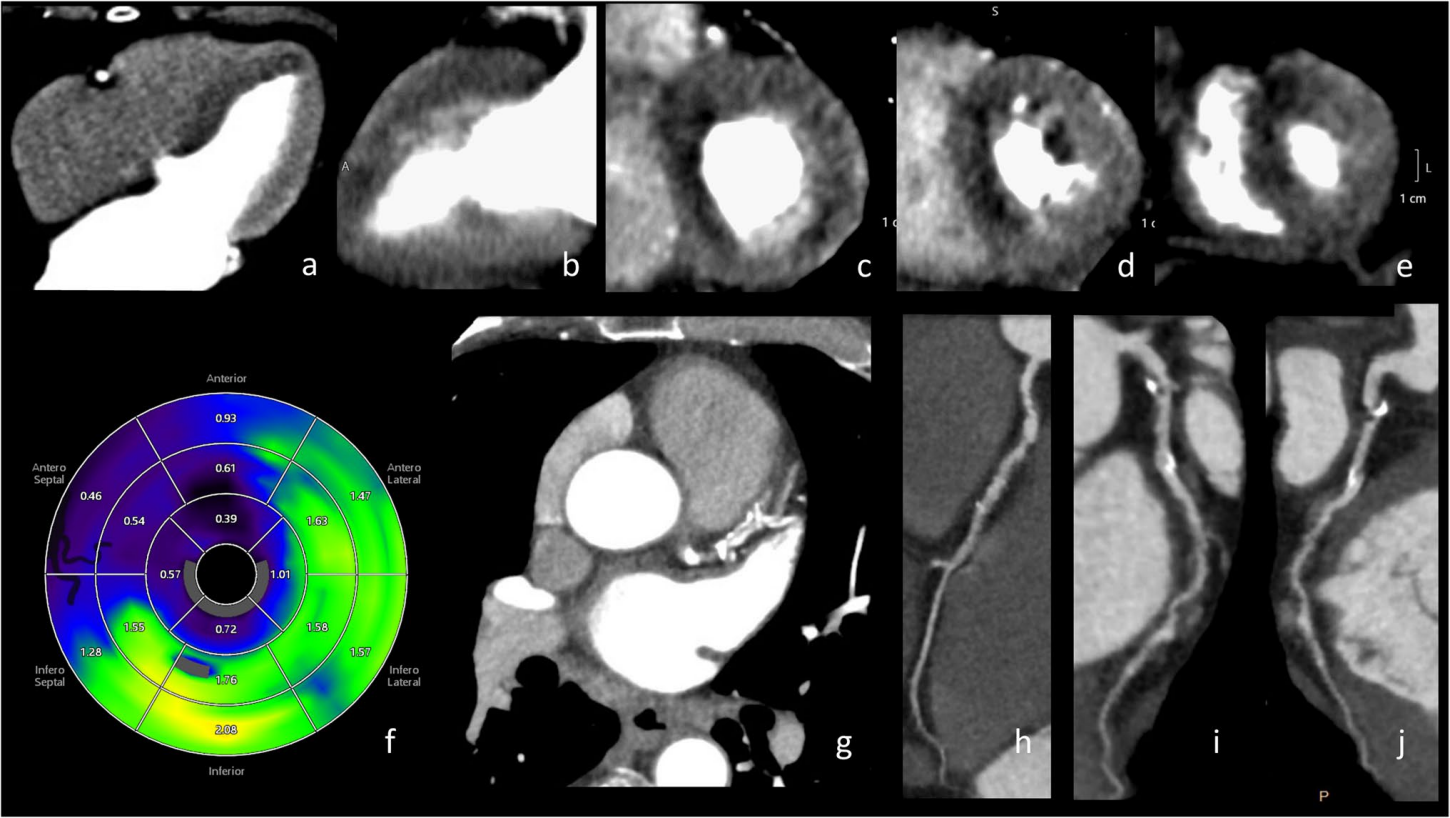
A 70-year-old male with poorly-regulated hypertension presented to the outpatient clinic with stable angina pectoris. Three months prior, he experienced chest pain, but acute coronary syndrome was excluded at the ER. The patient underwent a CT examination with calcium score, coronary CTA, and dynamic stress CTP protocol. The calcium score was 278.83 (62^nd^ percentile). Coronary CT angiography showed 50% stenosis in the distal left main artery (i), stenosis of 70–99% in the proximal (i), the mid-LAD (i), and origo LCX (j). In the proximal RCA (h), the intermediate stenosis based on the non-calcified plaque was 50%-69%. In the mid-LAD and mid-LCX: plaques with high-risk plaque features (positive remodeling and hypo-attenuated plaques). Positive adenosine stress CT perfusion with extensive ischemia in LAD territory in multiple segments (a–f). Furthermore, the ischemia in the basal slice in antero/inferolateral segments could be related to the left main stenosis  >50% and significant stenosis in proximal LCX (c). Furthermore, diffuse low-stress MBF values in all segments, LAD territory most severely affected, supporting the diagnosis of multiple vessel disease. The patient underwent invasive angiography that confirmed significant multiple vessel disease in LAD (proximal and mid-segment,  >70% stenosis) and proximal LCX (>70%). The intermediate lesion in the RCA was FFR-negative (FFR 0.83). The stenosis in the distal left main was 50%. The patient was referred for CABG. Upper row: dynamic myocardial CTP imaging: a four-chamber view, b two-chamber view; short axis: c basal view, d midventricular view, e apical view. Lower row: f dynamic CT perfusion map, g coronary CTA slice with LAD stenotic changes, h coronary CTA RCA, i coronary CTA LAD, and j coronary CTA LCX
